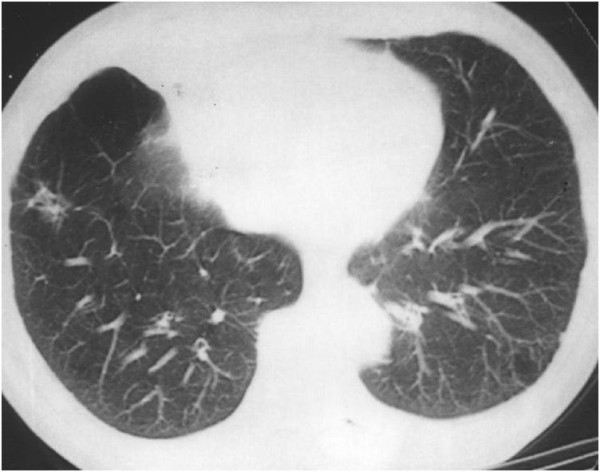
CT scan showing the absence of both lung metastases, which regressed after radiation therapy.
